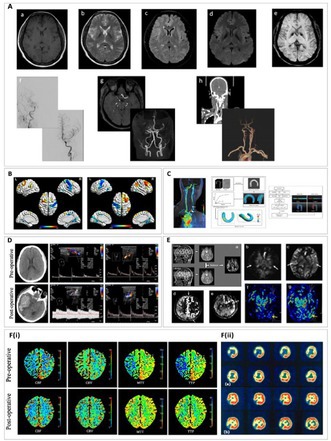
FIGURE 2 Multimodal clinical imaging approaches for evaluating cerebrovascular structure, perfusion, and hemodynamics in Moyamoya disease. Shown are representative MRI sequences and angiographic techniques (DSA, TOF‐MRA, CTA), functional MRI, 4D flow MRI.
